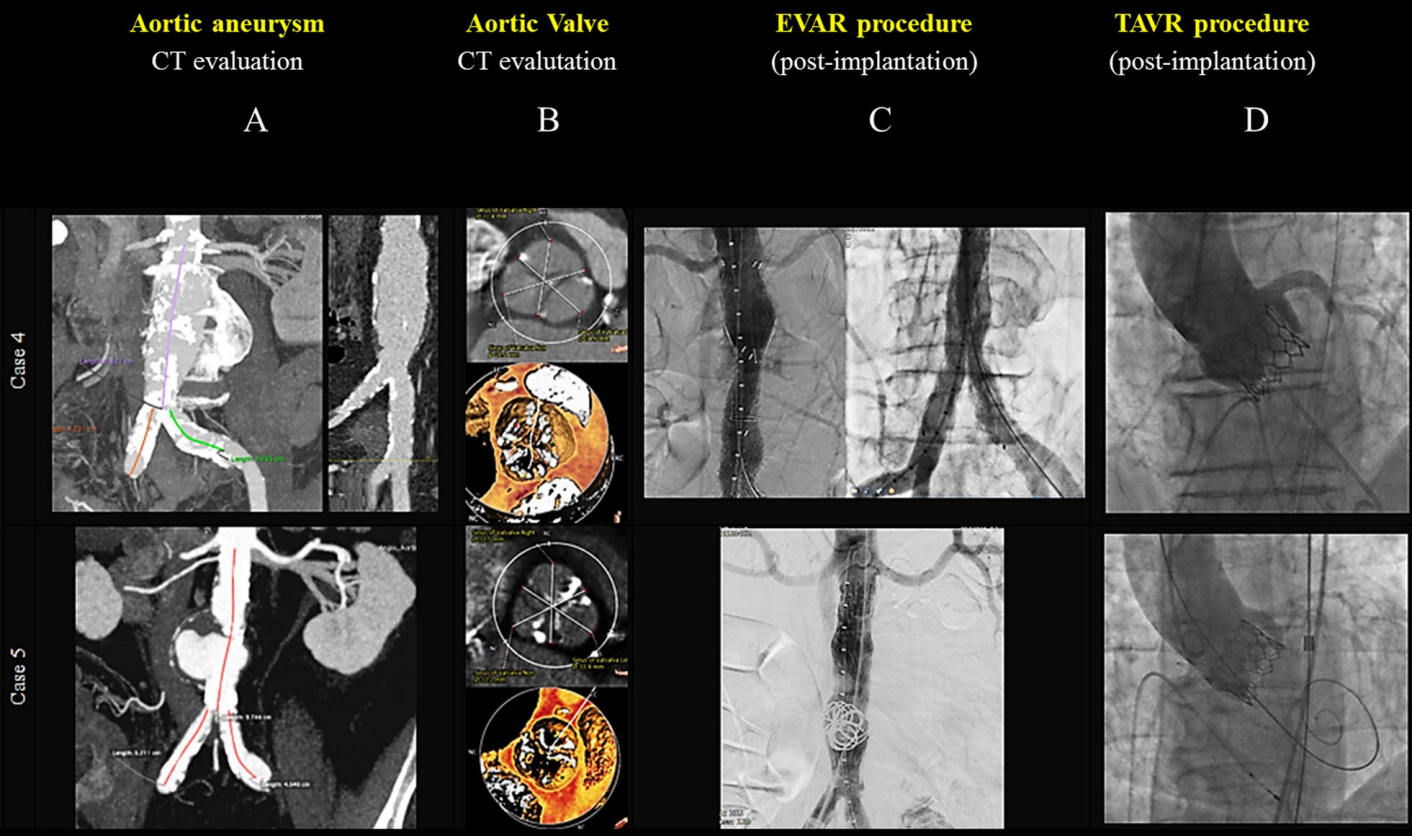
Cases 4–5 pre-procedural images of computed tomography (CT) angiography with tridimensional reconstruction of abdominal aortic aneurysm (panel A) and anatomy of the aortic valve (panel B). Post-procedural EVAR (panel C) and TAVR (panel D) results
